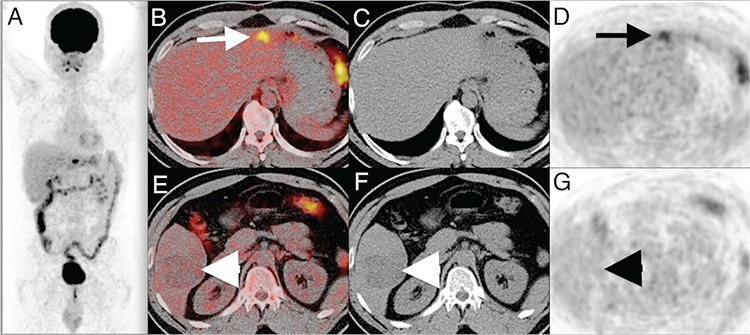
Positron emission tomography/computed tomography (PET/CT) images were acquired after a long period of fasting and 370 MBq (10 mCi) ^18^F-FDG administration. Maximum intensity projection (A) and axial slices of fusion (B, arrow), CT (C) and PET (D) images showed focal hyper-metabolic lesion in the left lobe, segment 2. A mass lesion, approximately 7 cm in diameter, with heterogeneous density at the right liver lobe segment 5 was detected, that displayed normal ^18^F-FDG uptake levels as the liver parenchyma (E arrowhead, F arrowhead, G). PET/CT did not reveal any other focus that was suspicious for malignancy. Hepatocellular carcinoma (HCC) and cholangiocellular carcinoma (CCC) are both primary malignant liver tumors originating from the hepatocyes and bile duct cells, respectively. The incidence of HCC and CCC together is extremely rare (lower than 1% of all primary malignant liver tumors) (1). Synchronous HCC and CCC cases, some of them on the same segment, detected with CT or magnetic resonance imaging (MRI) studies have previously been reported (2,3). Besides, both HCC and CCC components present in the same tumor and hepatic stem cells differentiating to hepatocytes or cholangio cells have also been reported (3). To our knowledge, this is the first case to report synchronous primary malignant liver tumors in two distinct segments detected by ^18^F-FDG PET/CT.
